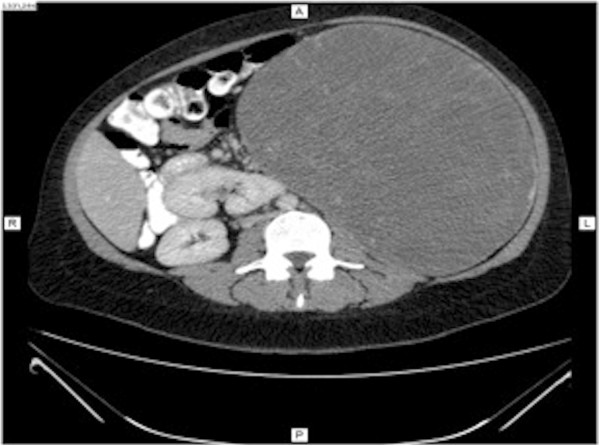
Computed tomography imaging of the splenic mass in venous phase.
